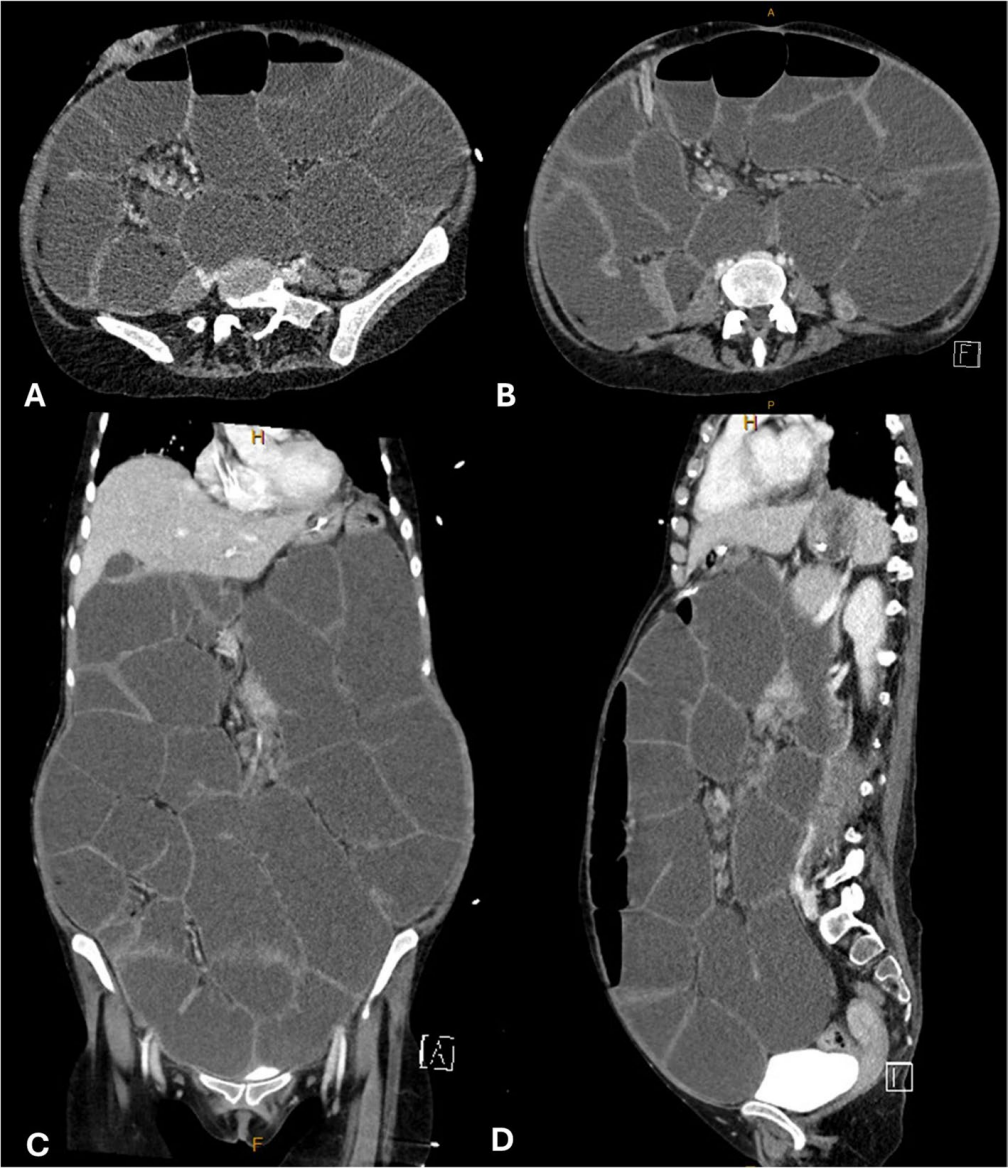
Abdominal Computed Tomography (CT) showing gastrointestinal ganglionosis with superimposed bowel obstruction in transverse (A and B), coronal (C) and sagittal views (D)
